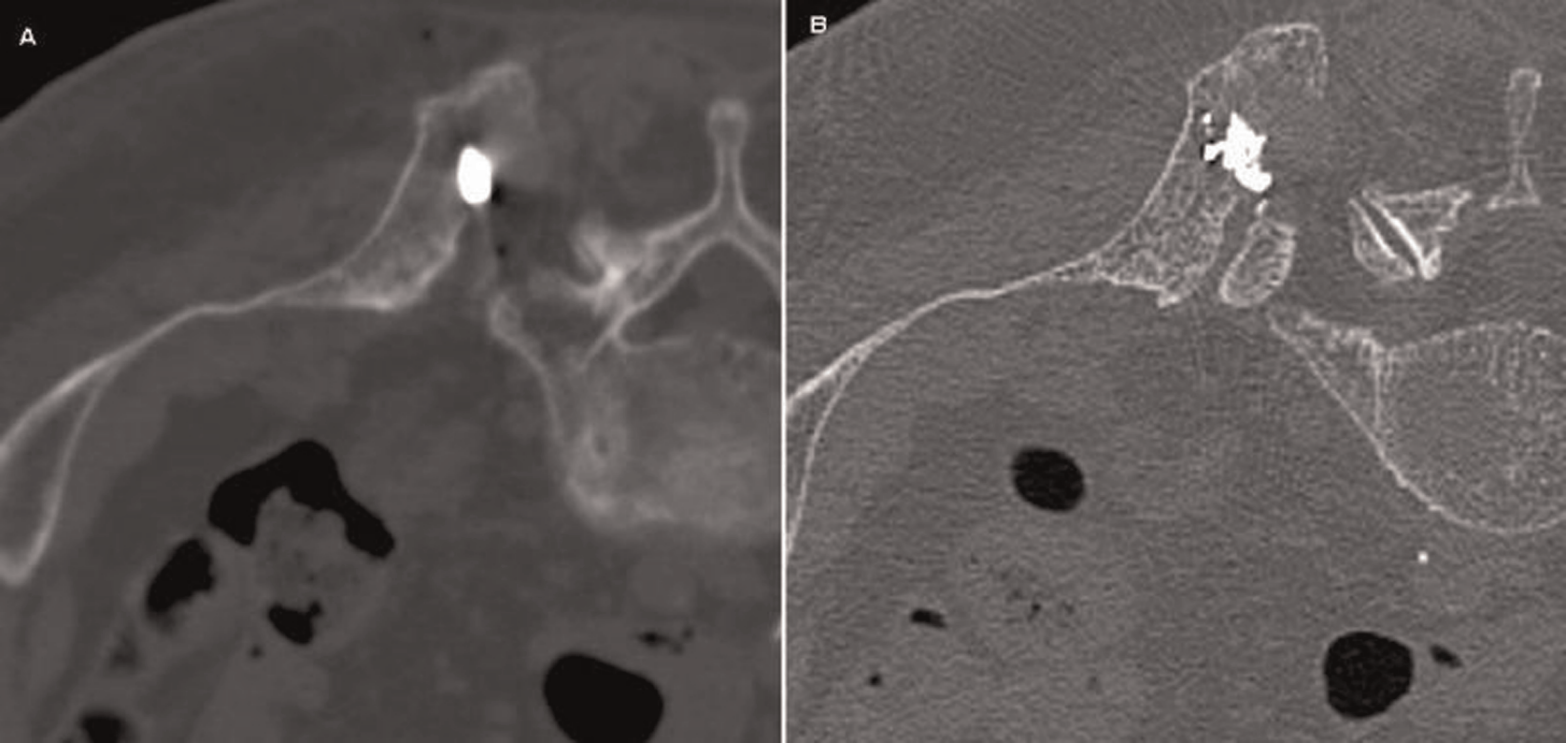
CT scan during the procedure. The electrode device is deployed inside the metastatic lesion and directed anteriorly through the malignant mass (A); after completing the ablation a mixture of bone cement and sterile barium sulfate was injected into the ablated cavity (B).
